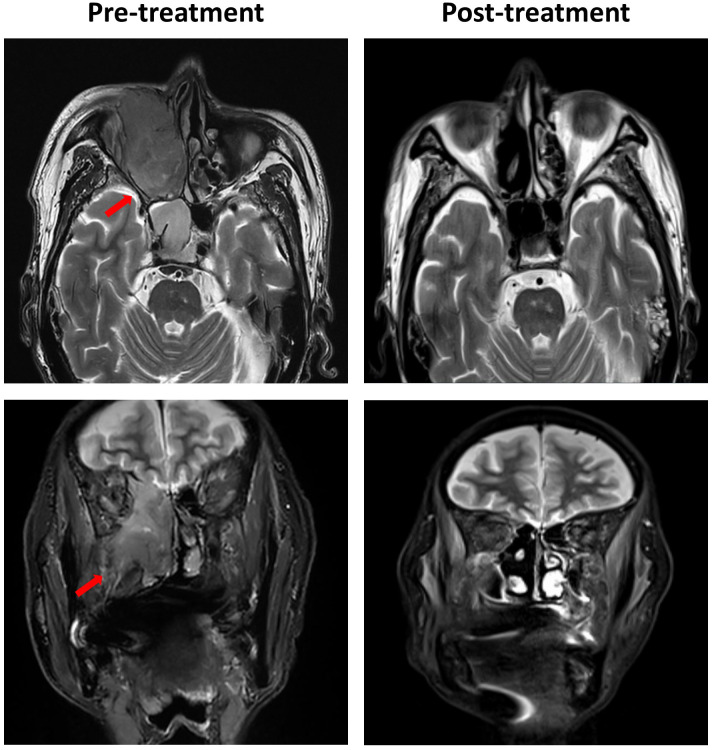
Nasopharynx MRI with contrast revealed tumor invasion pre and post immunotherapy and chemotherapy.
